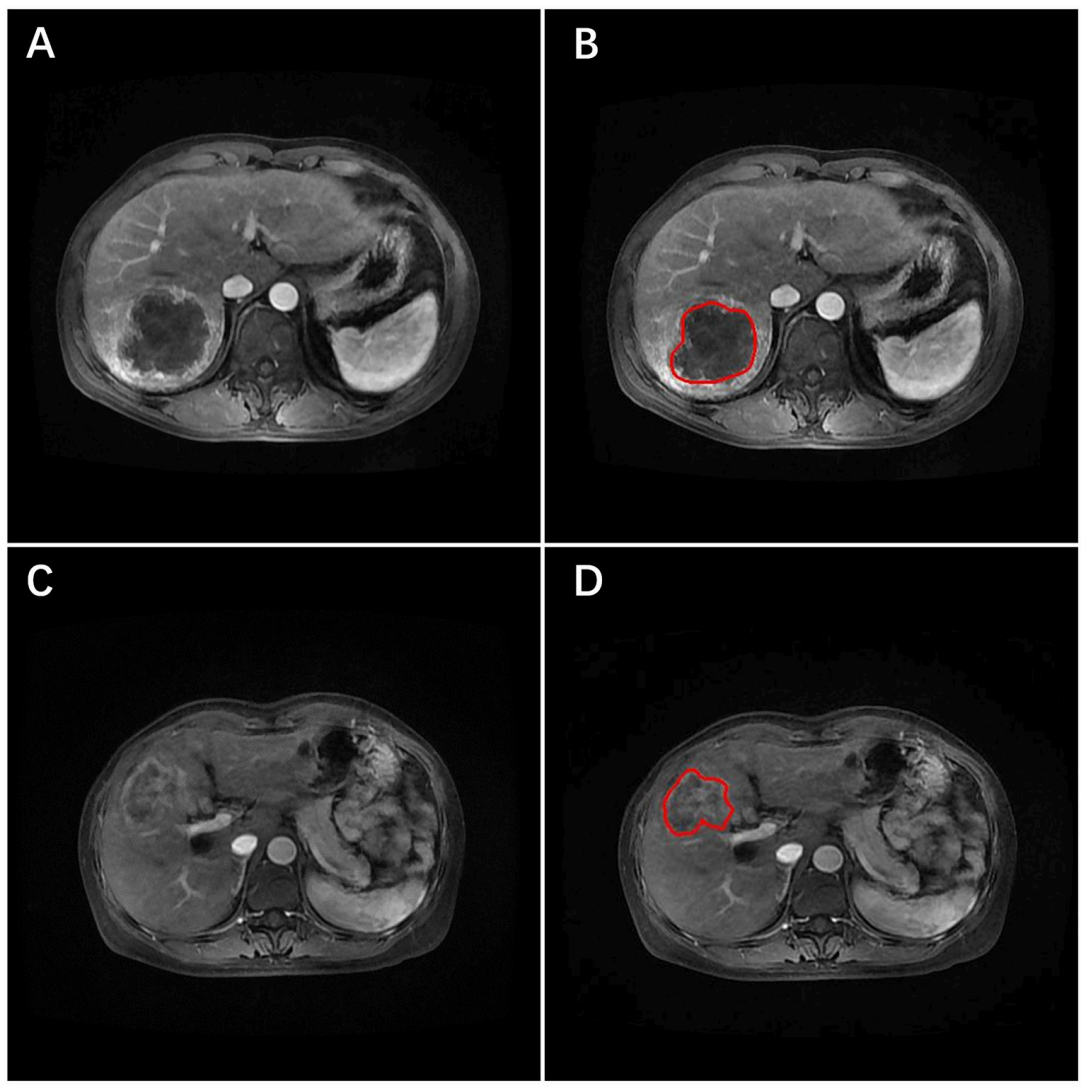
Arterial-phase contrast-enhanced MRI images of two patients with ICC. The tumor was identified, and the region of interest (ROI) placed (red line) on the images. (A,B) The primary image and the ROI (red line) marked image for one patient developed early recurrence. (C,D) The primary image and the ROI marked (red line) image for the other patient did not develop early recurrence.
